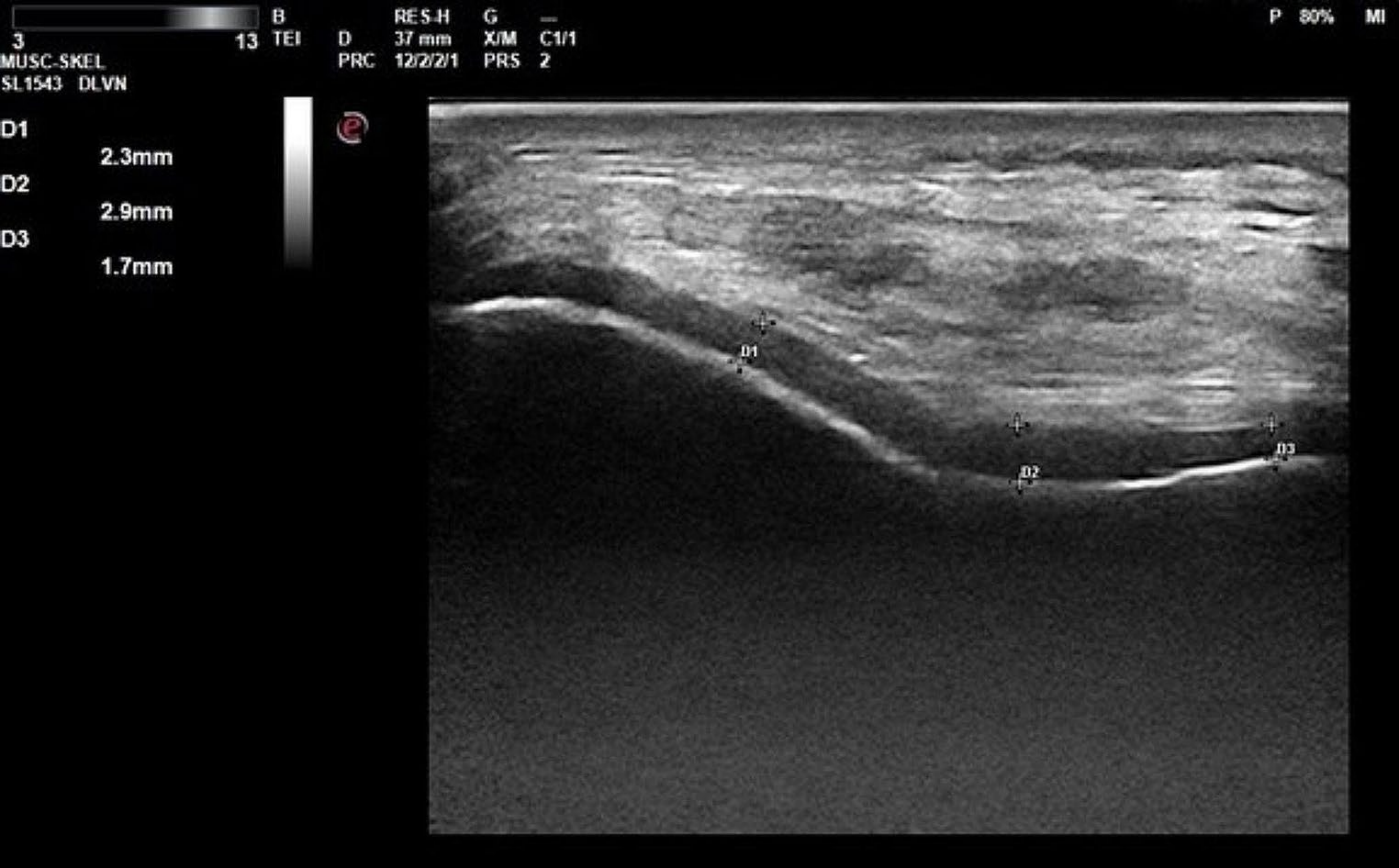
Ultrasonografic measurement of right distal femoral cartilage thickness. D1, right lateral condyle; D2, right intercondylar area; D3, right medial condyle
